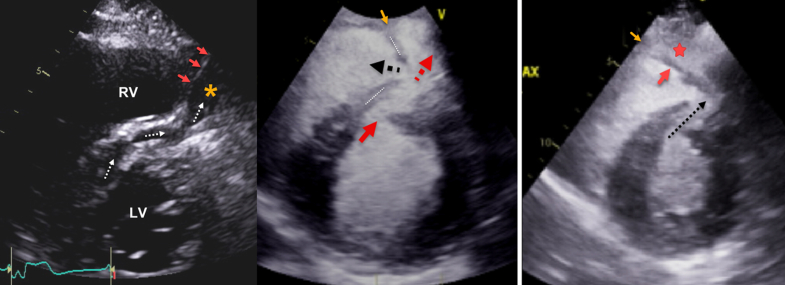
Parasternal Short-Axis Views (Left) Image without contrast shows VSD and septal dissection (dotted white arrows) that continues into an anterior RV dissection cavity (orange star) and begins at the anterior RV septal insertion point (red asterisk). The RV dissection cavity is demarcated from the RV cavity (red arrows). (Middle) Image with contrast shows the course of the VSD connection (red arrow) to the RV dissection cavity (dotted red arrow) and the outlet into the RV cavity (black arrow). The RV dissection cavity is demarcated anteriorly (orange arrow) from the RV. (Right) Image with contrast agent shows more extensively the flap of tissue (red arrow) that separates the RV from the RV dissection cavity (red star). The VSD and the septal dissection are also shown (black arrow). The RV dissection cavity is demarcated anteriorly (orange arrow) from the RV. RV = right ventricle; VSD = ventricular septal defect.
